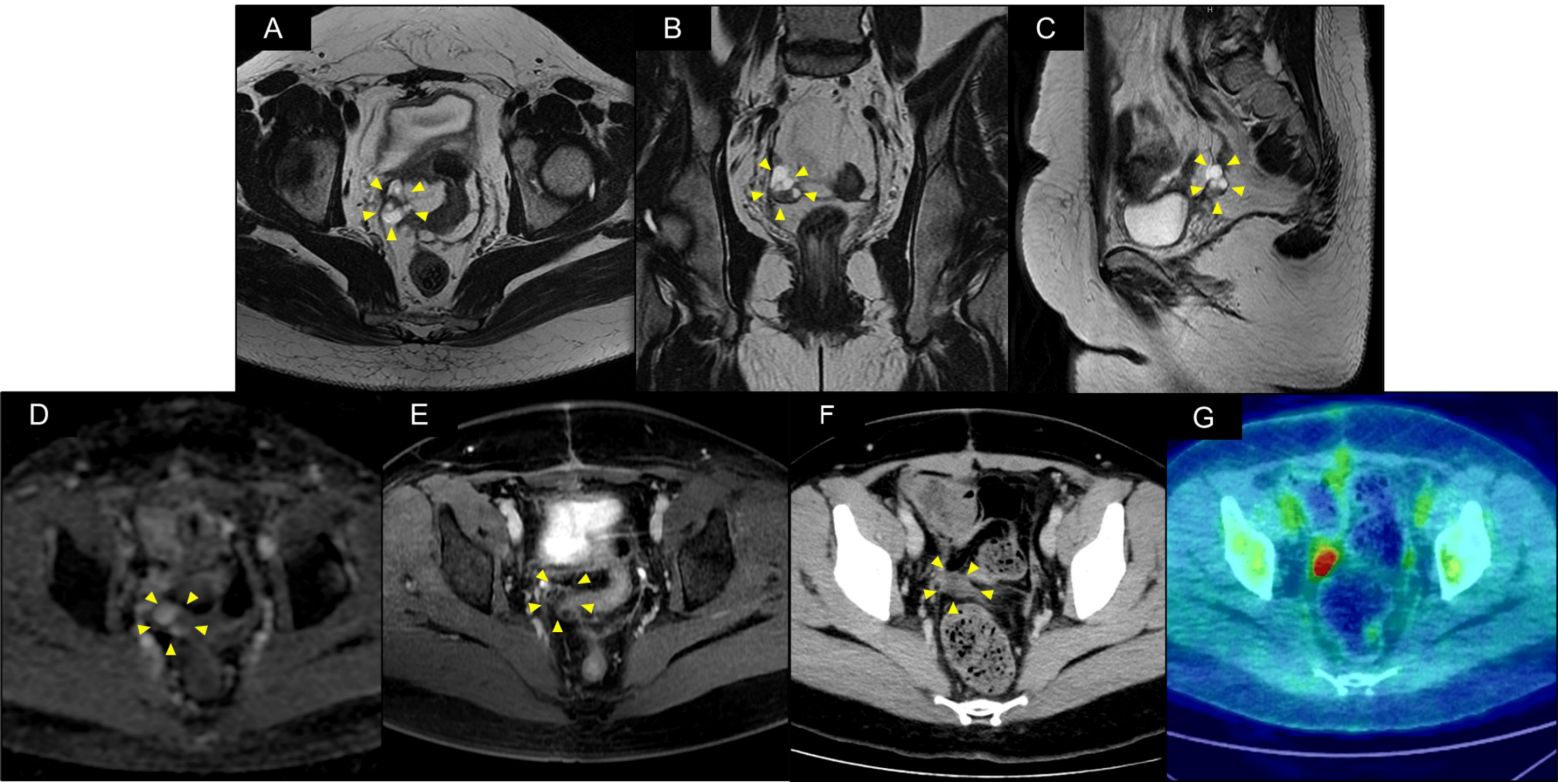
Pelvic imaging studies after four courses of postoperative chemotherapy. (A–C) Magnetic resonance imaging T2-weighted image axial, coronal, and sagittal cross-sections showing a mass with solid and cystic components on the right side of the rectum. (D) Apparent diffusion coefficient map demonstrating diffusion restriction in the solid component of the mass. (E, F) Contrast-enhanced T1-weighted image and contrast-enhanced computed tomography (CT) demonstrating delayed enhancement in the solid component of the mass. (G) Positron emission tomography/CT demonstrates ^18^F-fluorodeoxyglucose accumulation, with a maximum standardised uptake value of 25.65.
